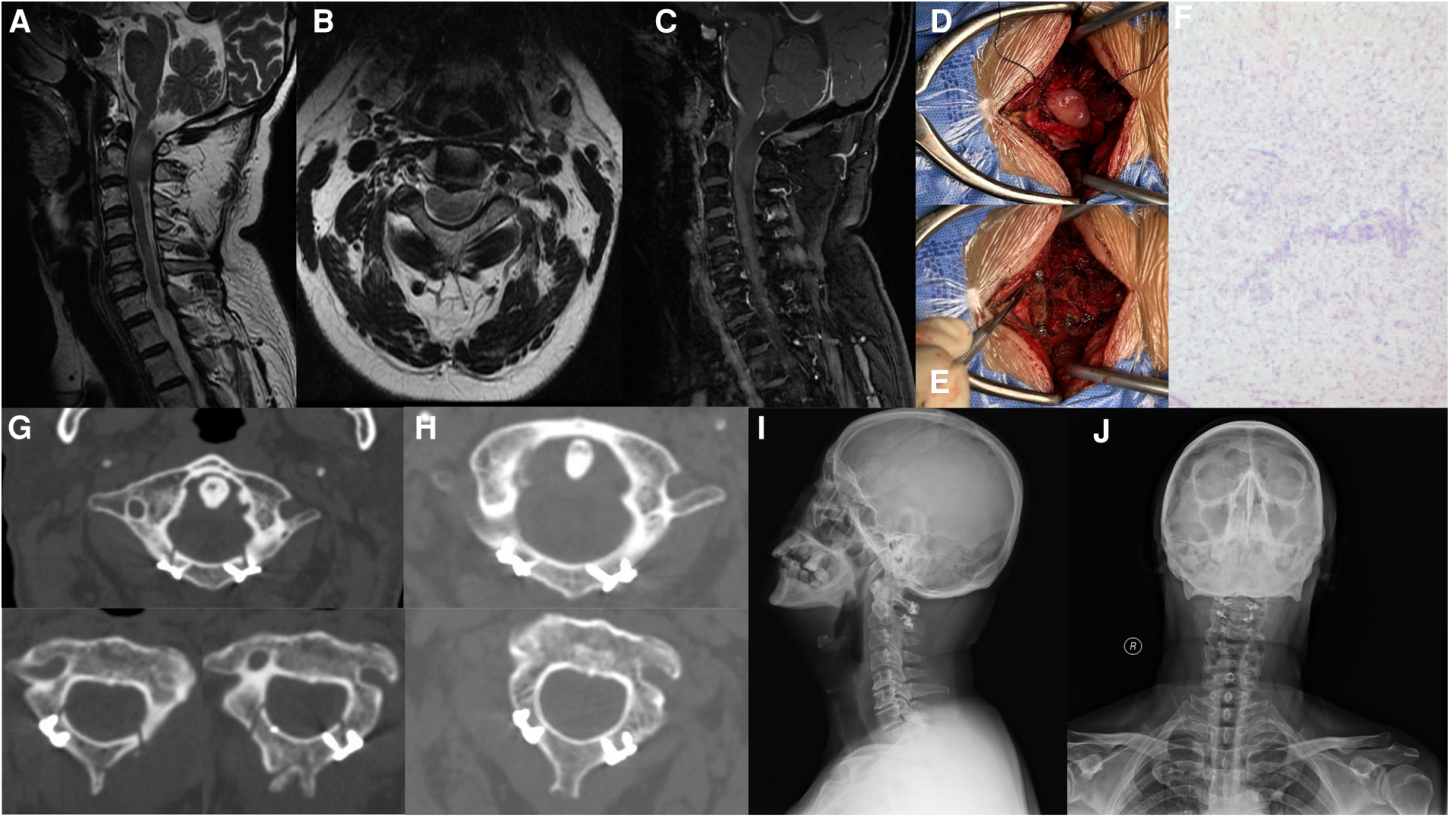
Case presentation. (A–C) Preoperative contrast-enhanced cervical Magnetic Resonance Imaging revealed an intradural-extramedullary mass measuring 3.7 × 1.7 × 3.0 cm, protruding outward through the left intervertebral foramen of C1-2. The lesion was of equal T1 and T2 signal with mild enhancement. (D) After removal of the posterior arch, bilateral axial lamina, and posterior ligamentous complex, a median linear incision of the dura was made to expose the tumor. (E) Following tumor removal, the posterior arch of the atlas and bilateral axial lamina were repositioned and fixed in their physiological location. (F) Postoperative pathology confirmed a diagnosis of Schwannoma. (G) Computed tomography (CT) conducted at 3 days after surgery showed satisfied position of the internal fixation. (H–J) Postoperative CT and plain radiograph revealed the progressive ossification of the posterior arch and axial lamina, with no signs of instability or deformities.
